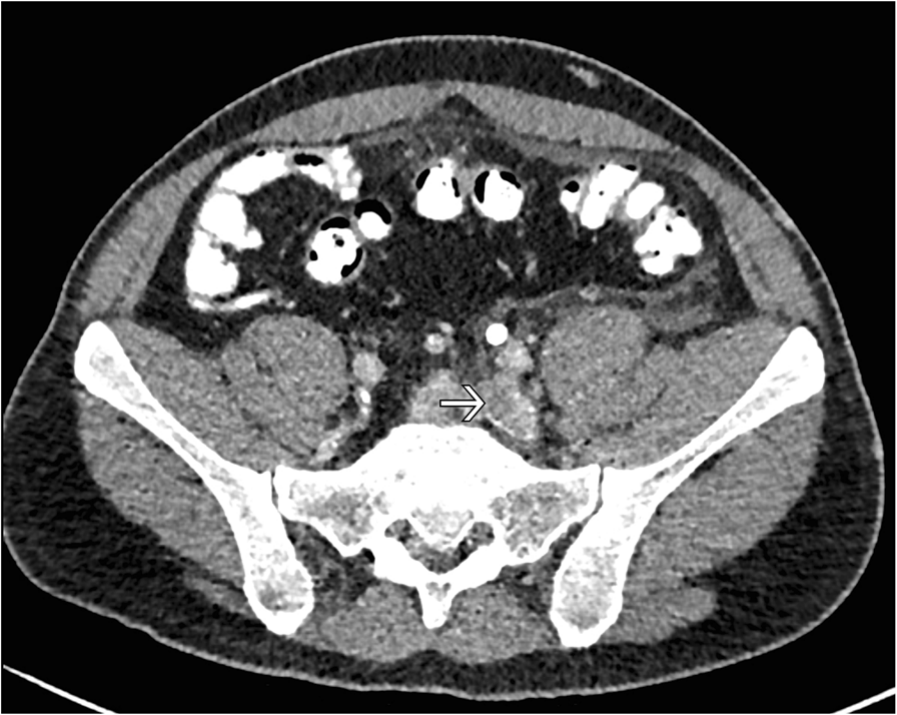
Axial abdominal CT Scan. CT shows left iliac vein thrombosis (arrow)
